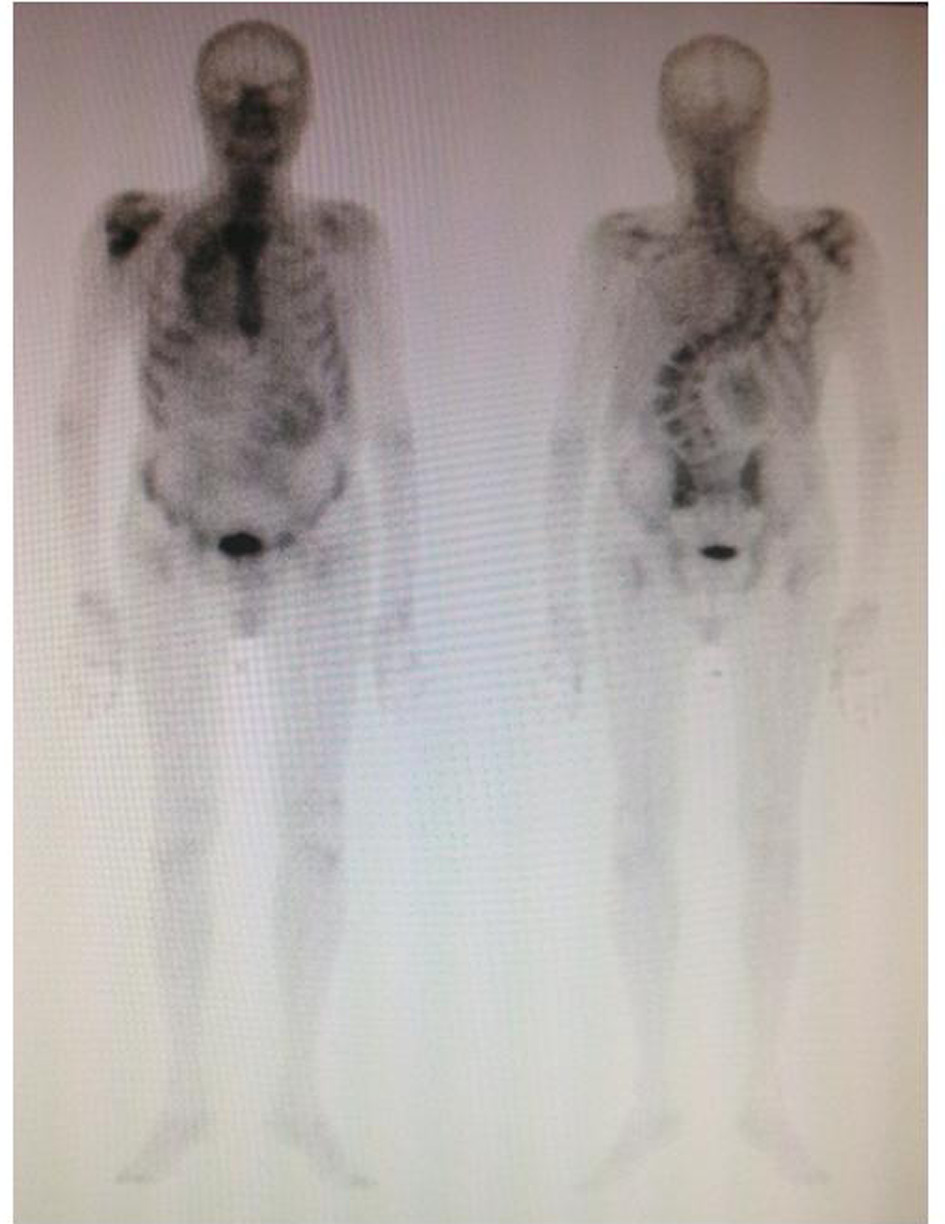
Total body bone scintigraphy showed increased activity in right humerus.
